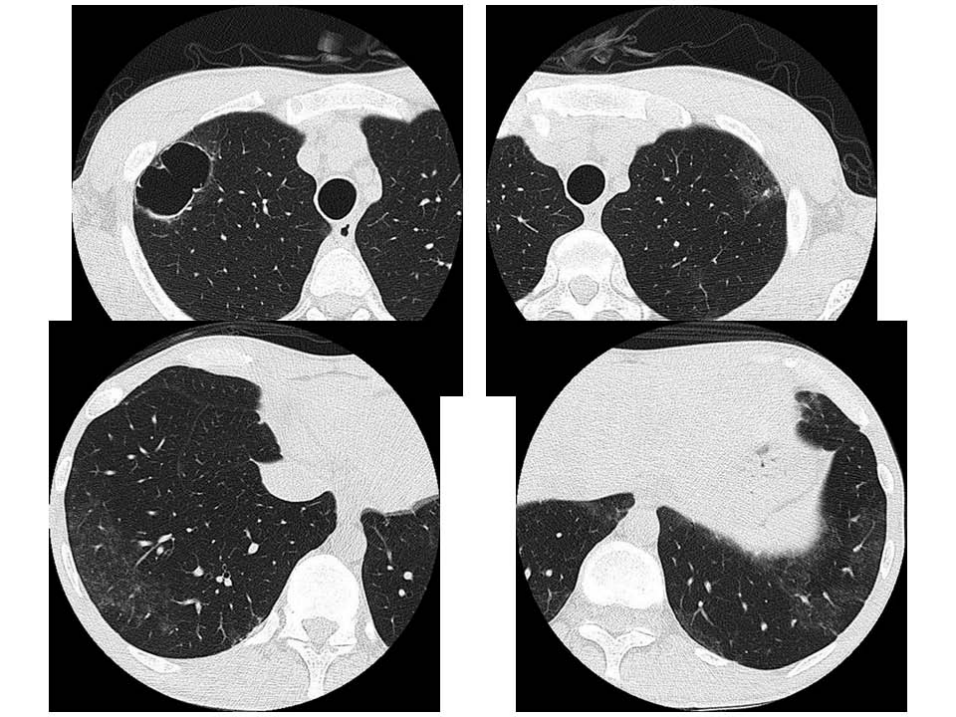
High-resolution computed tomography images of the lung 20 days after sulfamethoxazole trimethoprim treatment. Various sizes of cystic lesions are seen in the bilateral upper lobes and small nodules are seen in the bilateral lower lobes.
